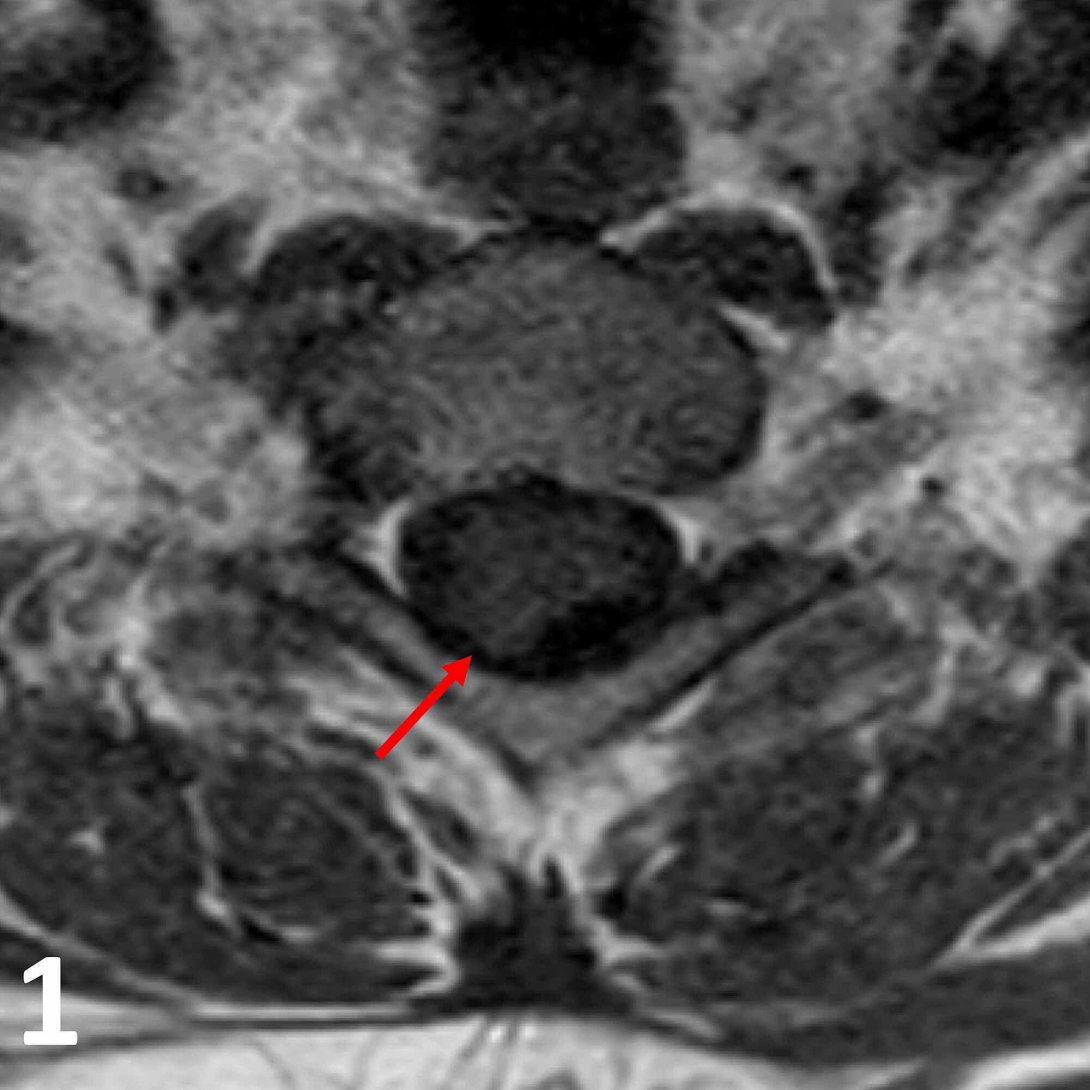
Axial T1 pre-contrast MRI image at the T1 vertebral level. Note the T1 isointense intradural lesion (red arrow) in the right posterolateral aspect of the spinal cord.
